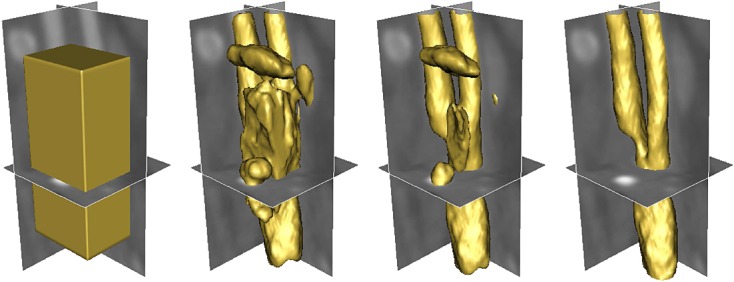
Segmentation of the carotid from computed tomography image using gradient vector interaction-based and shape-based energies.
